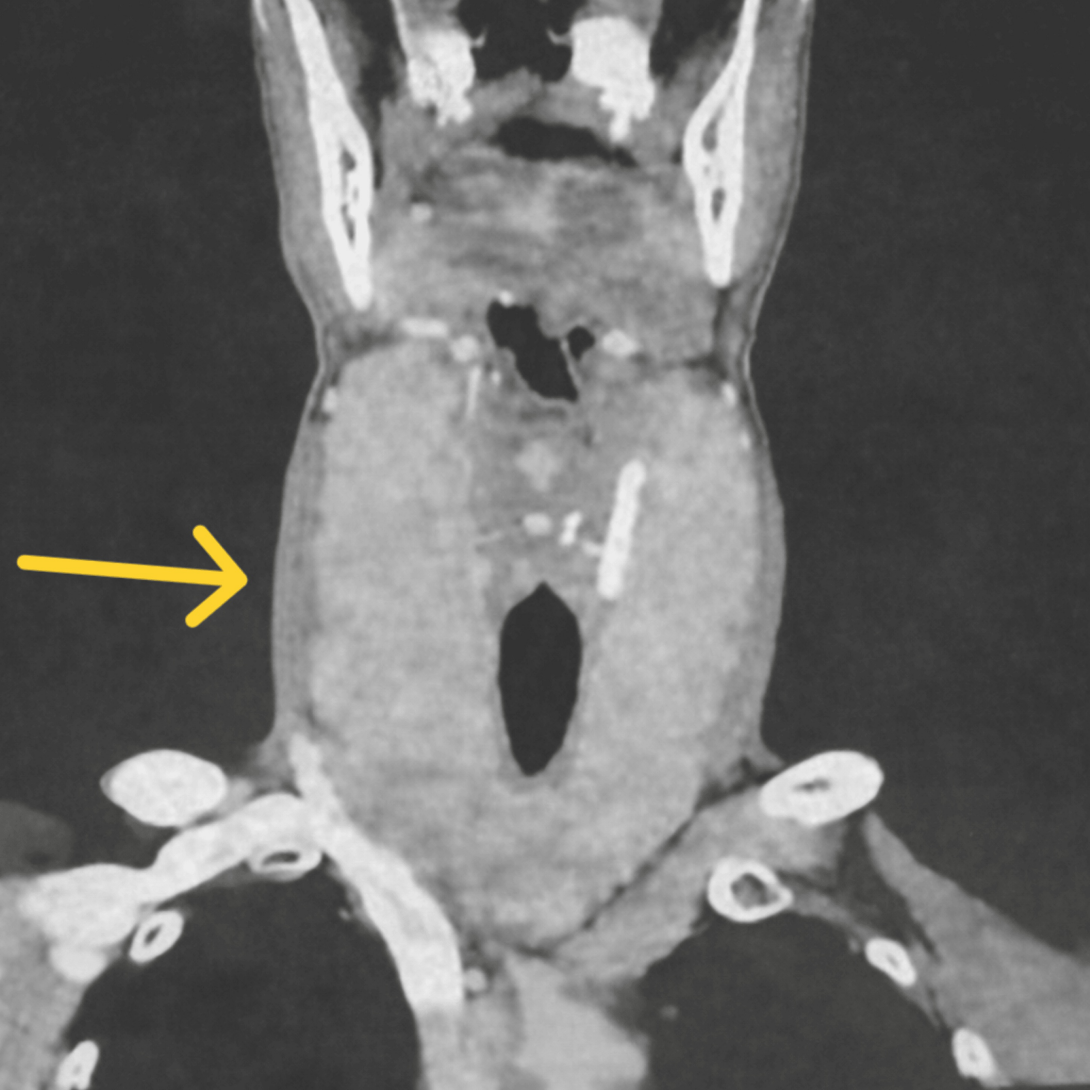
Cervicothoracic CT (frontal view) showing a large substernal goiter (yellow arrow).
